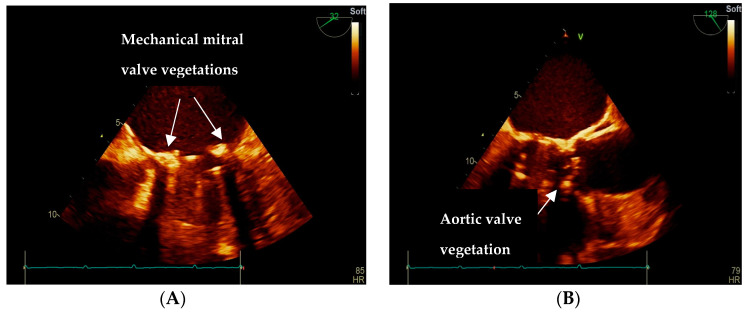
Transesophageal echocardiography. (A) Anterior and posterior mitral mechanical valve insertion vegetations. (B) Vegetation of the aortic non-coronary cusp (from Cardiology Clinic, “St. Spiridon” Emergency Clinical County Hospital, Iasi, Romania).
